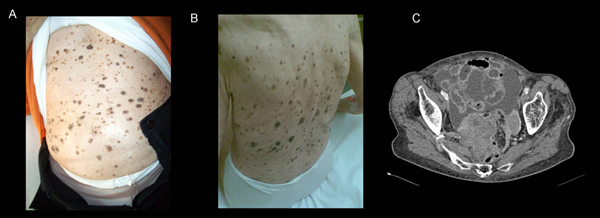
(A) and (B) Widespread seborrheic keratoses. (C) Computed tomography scan showing a necrotic tumor accompanied by signs of peritoneal carcinomatosis.
